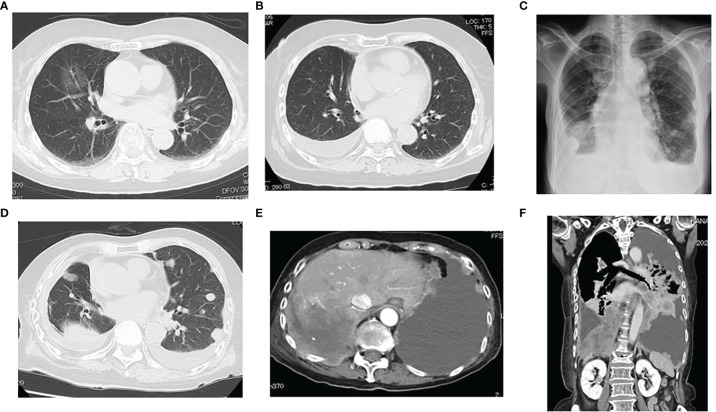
(A) Chest CT showing a ground glass opacity of 32 mm in size in her middle lobe of the right lung. (B) At 2 years and 3 months after pulmonary resection, the follow-up chest CT revealed right pleural effusion. (C–F) At 4 year and 7 months after pulmonary resection, the chest CT revealed multiple pulmonary nodules and bilateral pleural effusion with a right pleural tumor directly invading into the abdominal cavity. A right malignant pleural mesothelioma (sarcomatoid type) invaded to the right adrenal gland and the liver.
